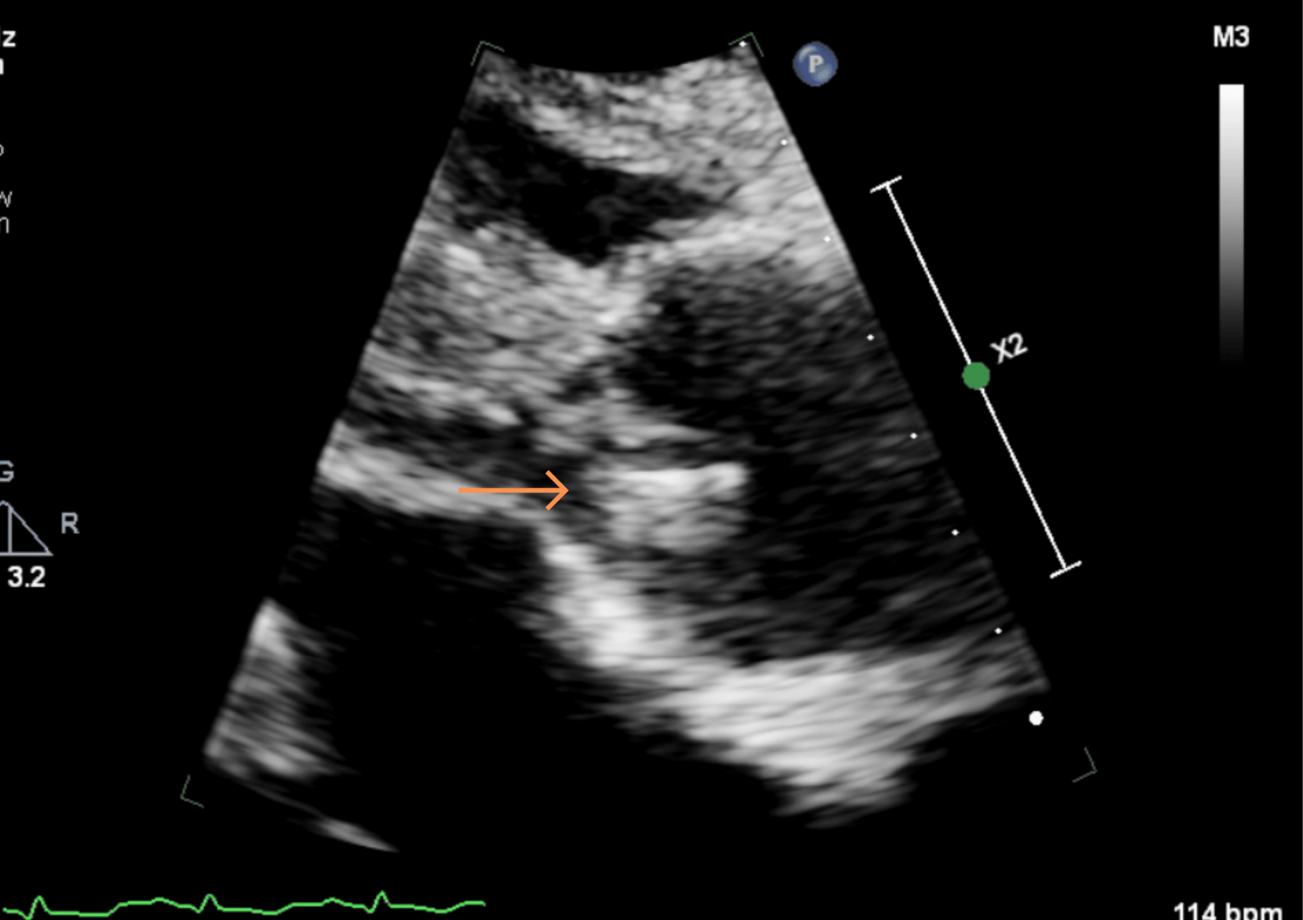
TTE PLAX view – zoom in on the aortic valve (orange arrow) TTE: Transthoracic echocardiogram, PLAX: Parasternal long axis
